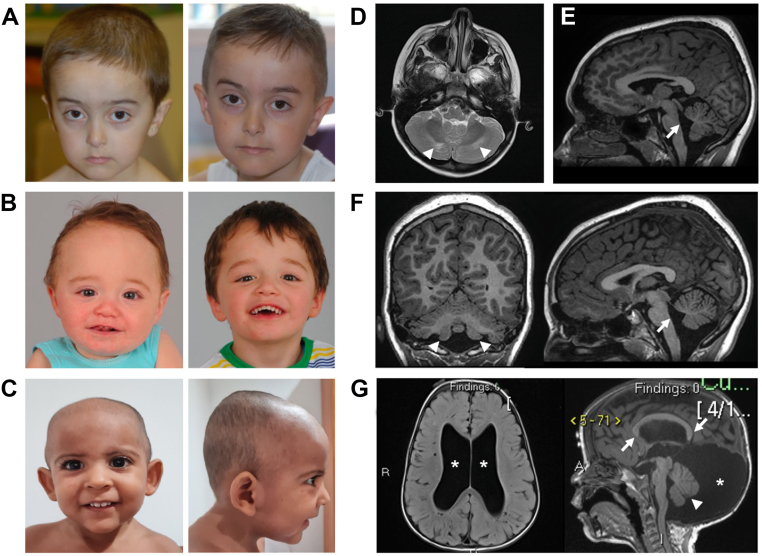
Facial features and structural brain anomalies in individuals heterozygous for ZIC1 variants. A. Facial appearance of proband from family 6 aged 6 years (left) and 8 years (right). B. Facial appearance of proband from family 17 aged 14 months (left) and 3 years 9 months (right). C. Facial appearance of proband from family 11 aged 19 months. D. Axial T2-weighted MRI brain section through the cerebellum of proband from family 1 aged 3 years demonstrating bilateral cerebellar hyperintensity at the gray-white matter junction (arrowheads). E and F. MRI brain images of individuals from family 2. E. Midline sagittal MRI of II-2 aged 14 years demonstrating mild cerebellar atrophy, thin brainstem and dilatation of the 4th ventricle (arrow). F. Coronal (left) and sagittal (right) MRI brain images of II-1 aged 8 years demonstrating hypoplasia and symmetrical dysplasia of the cerebellar hemispheres most evident inferiorly (arrowheads), with thin brainstem and 4th ventricle dilatation communicating with an enlarged posterior fossa (arrow). Both II-1 and II-2 also had white matter hyperintensities on MRI FLAIR imaging. G. MRI images of proband from family 7 aged 23 months. Left: Axial FLAIR MRI image demonstrates mild periventricular white matter loss, prominent perivascular spaces, and mild to moderate supratentorial ventriculomegaly with colpocephaly of the lateral ventricles (stars). Right: Sagittal T1-weighted MRI demonstrating large cyst of the posterior fossa (star), pontocerebellar hypoplasia (arrow head) and partial agenesis of the corpus callosum with hypoplasia of the rostrum and splenium (arrows).
